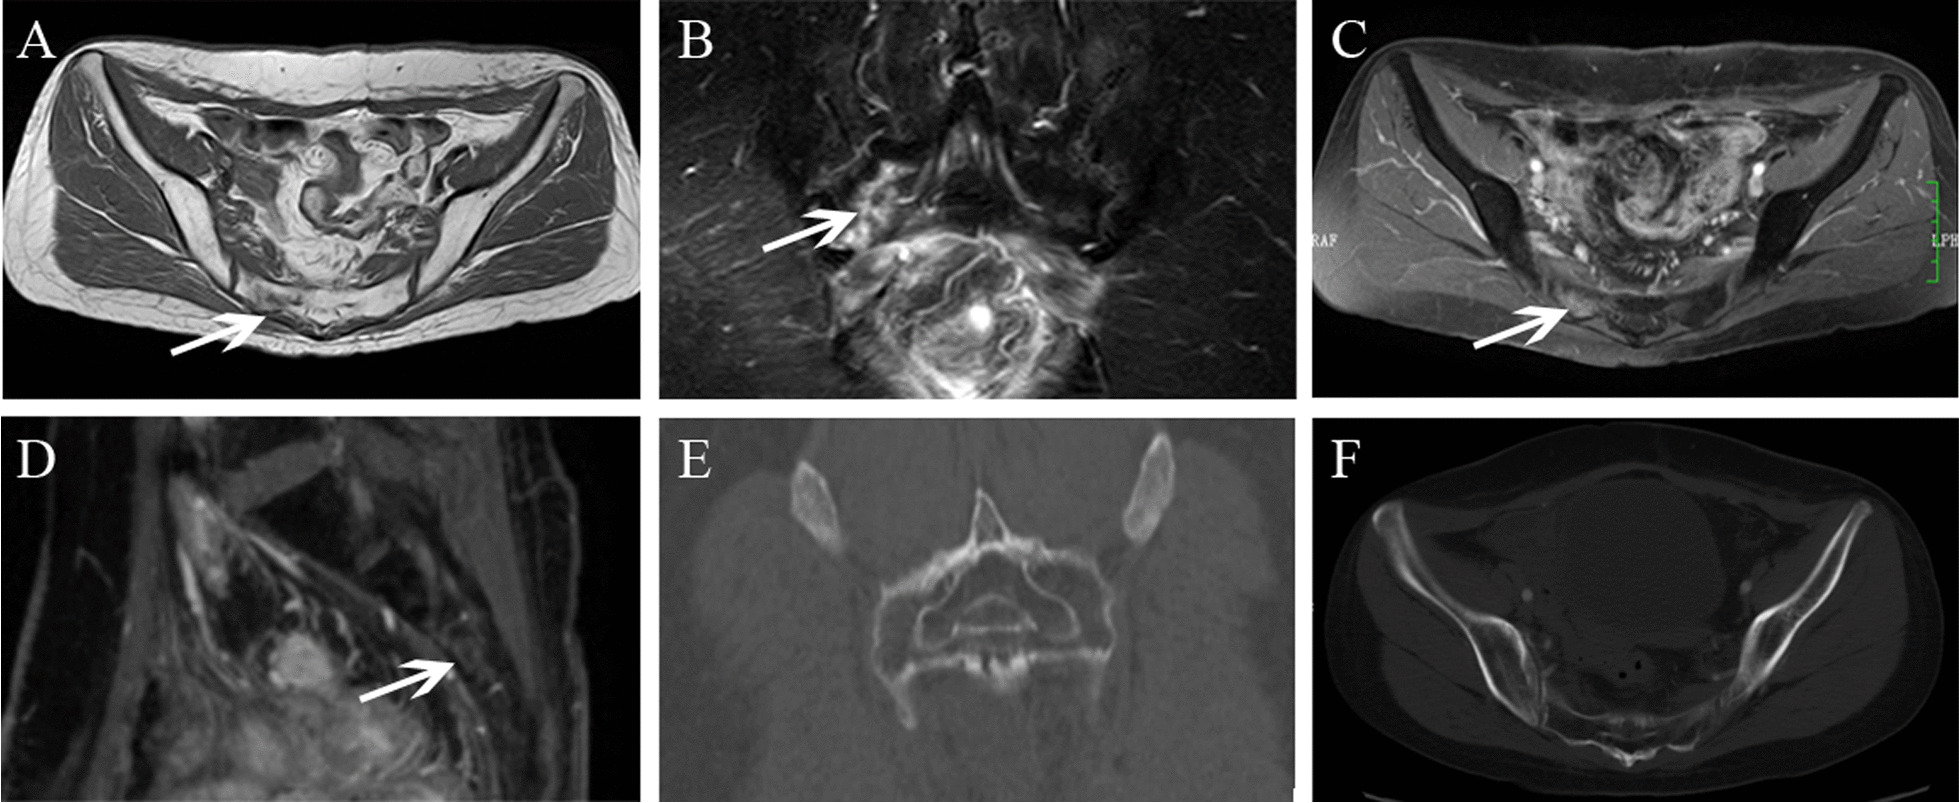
Right SIF in a 65-year-old woman with cervical cancer after radiotherapy. A Axial T1WI showed hypointensity. B Coronal FS-T2WI showed severe bone marrow edema with fracture line (arrow). C, D Axial and sagittal enhanced T1WI showed contrast enhancement. E, F Coronal and axial CT images showed no overt positive finding
